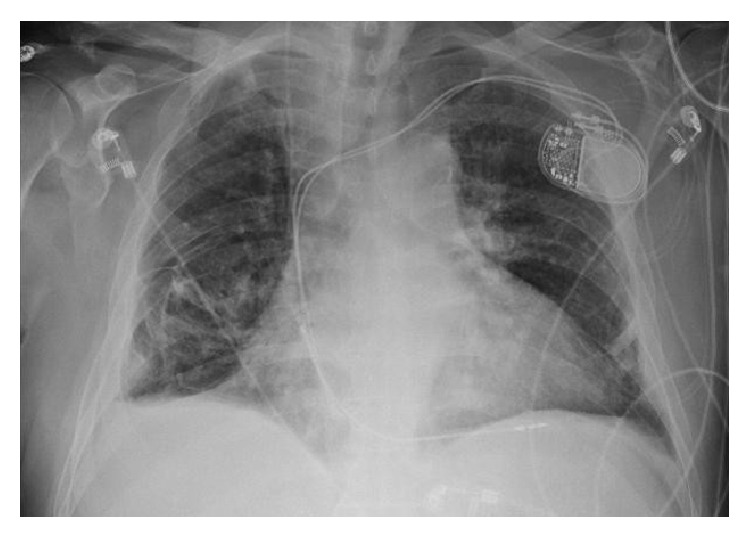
Follow-up chest X-ray showing improvement of lung consolidations.
